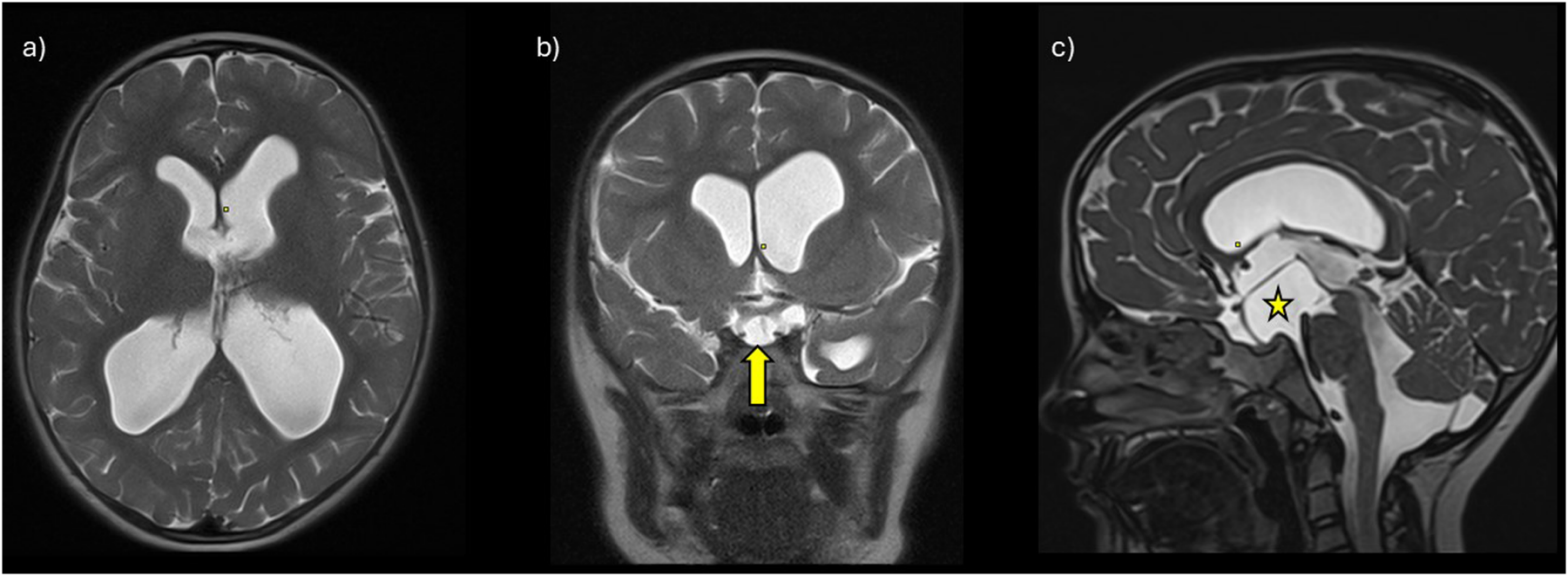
Brain magnetic resonance imaging (MRI) findings of the reported patient. From left to right (a-c): axial, coronal, and sagittal T2-weighted data. Different magnetic resonance imaging (MRI) sequences were used (turbo spin-echo [TSE] in a and b, SPACE [Sampling Perfection with Application optimized Contrast using different flip angle Evolution] in c). Ventriculomegaly is visible in all planes (a-c). The yellow arrow and the star indicate the arachnoid cyst in the interpeduncular cistern (b-c), with mass effect on the optic chiasm and optic nerves (b), and on the pituitary stalk, mamillary bodies, and the midbrain (c). Flow artifacts of the cerebrospinal fluid can be seen in the foramen of Monro on both sides (a) and in the cerebral aqueduct (c).
